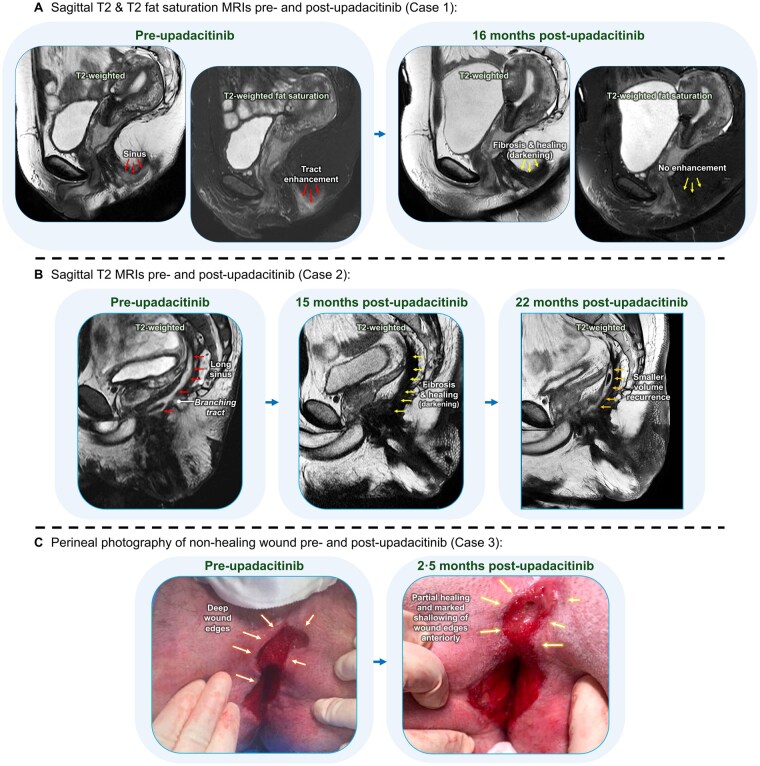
Magnetic resonance imaging (MRI) and perineal photography demonstrating perineal disease pre- and post-upadacitinib.
